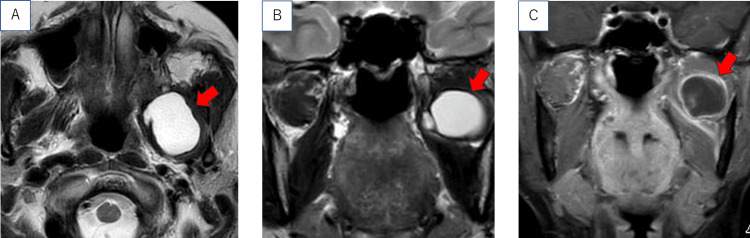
MRI images taken during the first consultation at our hospital. (A) T2-weighted horizontal section. (B) T2-weighted coronal section. (C) Contrast-enhanced T1-weighted coronal section. The red arrow indicates the lesion.
